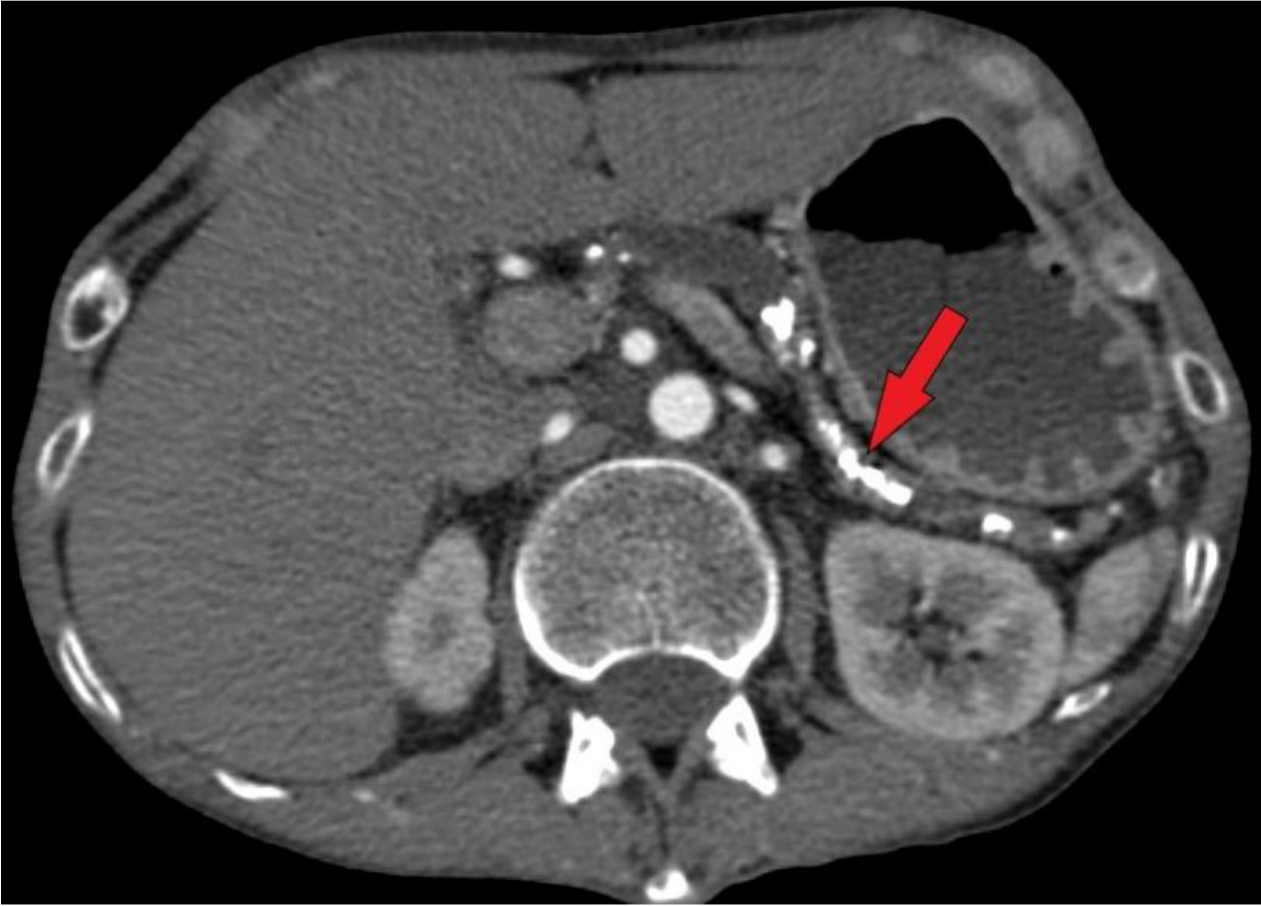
Axial plane of contrast-enhanced abdominal and pelvic CT demonstrates multiple pancreatic calcifications (red arrow), along with dilation of the pancreatic duct measuring up to 0.8 cm. These findings are consistent with chronic pancreatitis. Image courtesy of the Radiology Department, Kaunas Clinics
